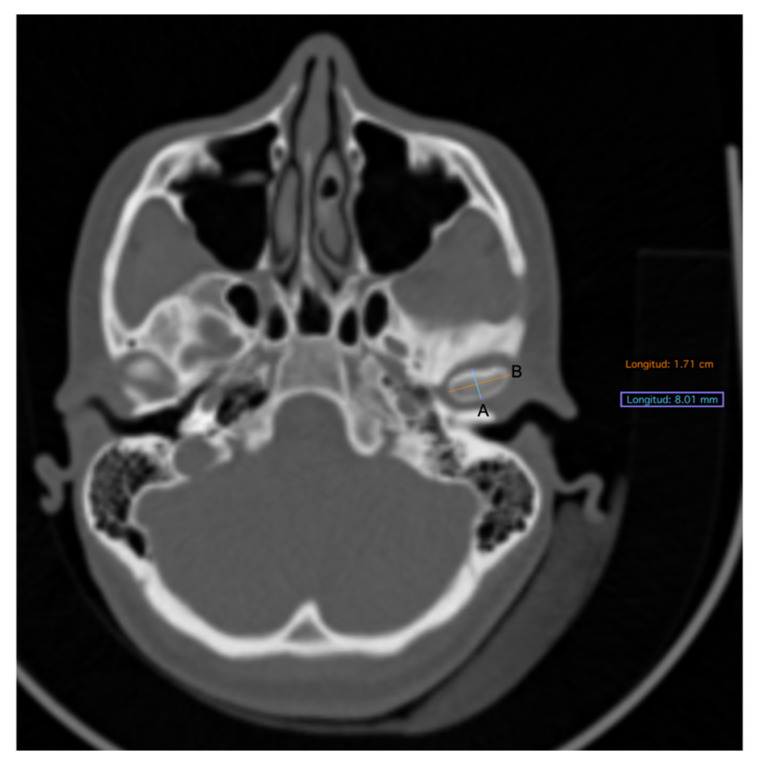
Bone tissue CT, axial view. (A) Anteroposterior condylar length. (B) Midlateral condylar length.
